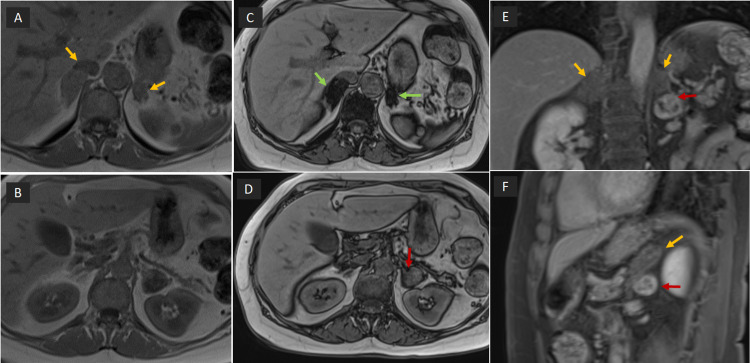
MRI abdomen (A) and (B) in phase, (C) and (D) out of phase images: Both adrenal glands are bulky (orange arrow), measuring 2.9 x 2 cm on the right and 2.5 x 1.5 cm on the left, with significant signal drop-off (green arrow) suggestive of adenomatoid hyperplasia. Exophytic left adrenal gland lesion does not show signal drop out (red arrow).  (E) and (F): Post-contrast Coronal and Sagittal images - no significant enhancement of bulky adrenal glands (orange arrow), heterogeneous enhancement of exophytic left adrenal gland lesion (red arrow).
